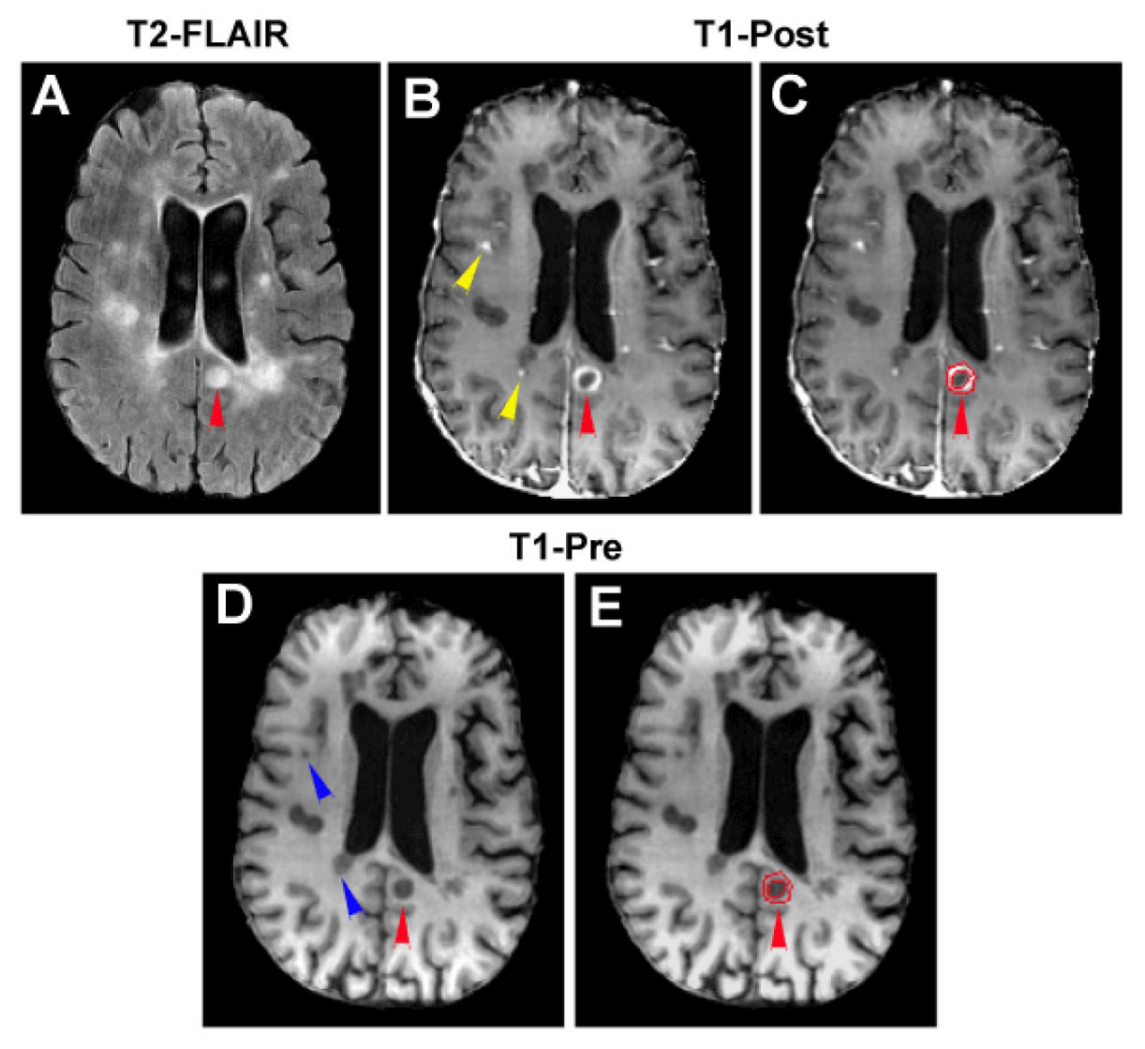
An axial section of T2-FLAIR (A), T1-Post (B and C) and T1-Pre (D and E) image showing a typical ring-enhanced lesion, its corresponding ROIs and the spatial relationship between active and T1-hypointense lesion. A hyperintense spot observed on T2-FLAIR image (red arrowhead in A) may represent a ring-enhanced lesion on T1-Post image (red arrowheads in B and C). It should be noted that remarkable T1 signal loss occurred inside the enhanced ring (E). The entire lesion area was then divided into two regions, T1-enhanced (C, the area encompassed by two red tracks) and T1-hypointense (C, the rest central part encompassed by the smaller red track) regions. Just like the ring-enhanced region surrounding the hypointense area, some active lesions appeared immediately adjacent to the T1-hypointense lesions (comparing the locations of enhanced regions indicated by yellow arrowheads in B and the locations of hypointense areas indicated by blue arrowheads in D).
